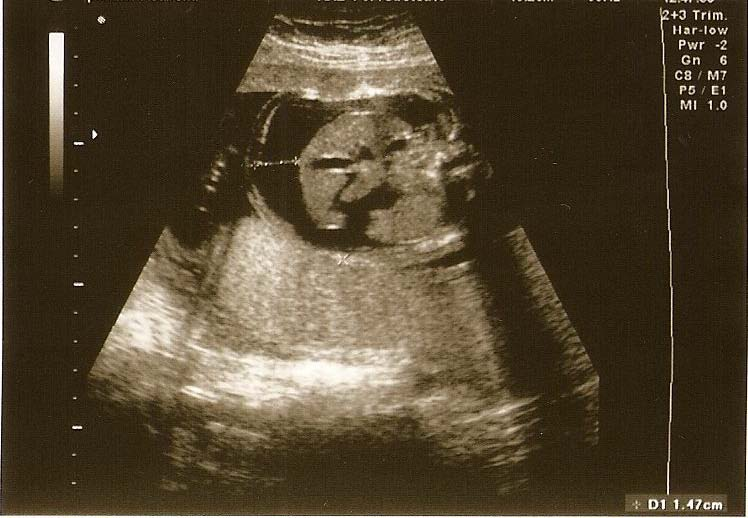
Sonogram showing fetal ascites
